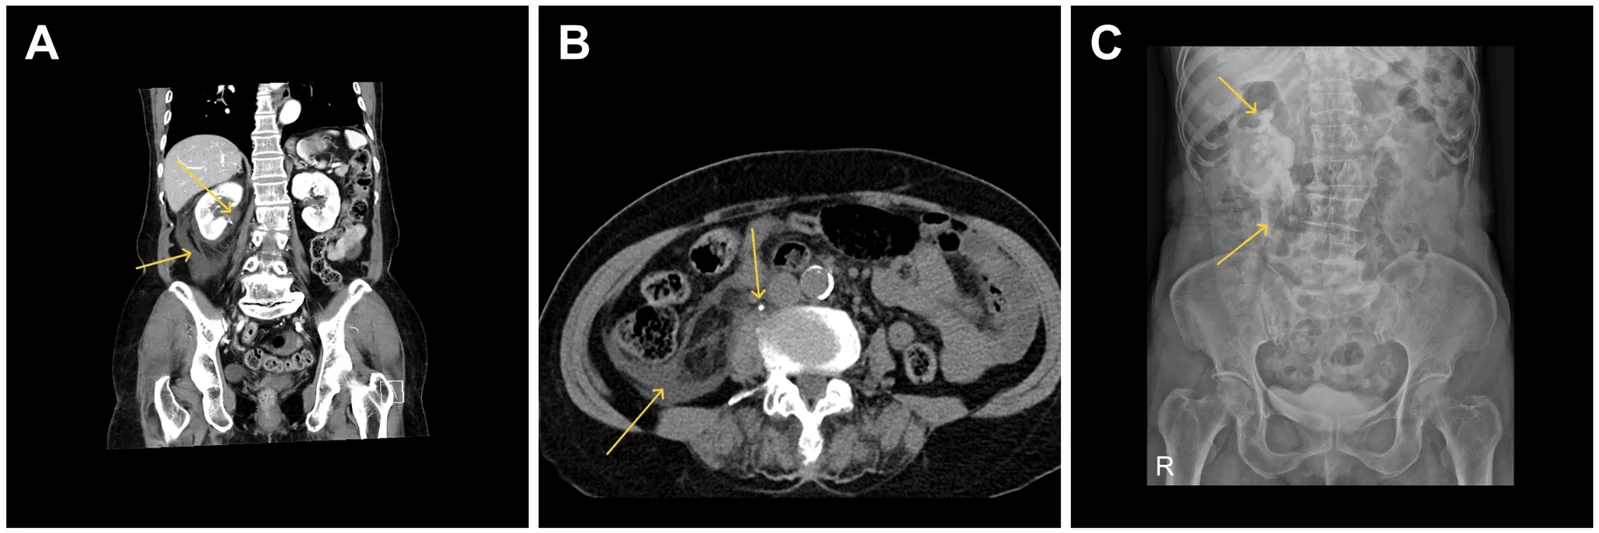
Imaging at presentation confirming forniceal rupture with retroperitoneal urine leakage. (A) Coronal contrast-enhanced computed tomography (CT) showing right hydronephrosis (upper arrow) and perirenal fluid (lower arrow). (B) Axial contrast-enhanced CT showing the obstructing right ureteral stone (upper arrow) and adjacent retroperitoneal fluid (lower arrow). (C) Post-CT plain radiograph (kidney-ureter-bladder) showing contrast-medium spillage in the right upper abdomen (arrows), the direct radiographic sign of forniceal rupture with retroperitoneal urine leakage.
